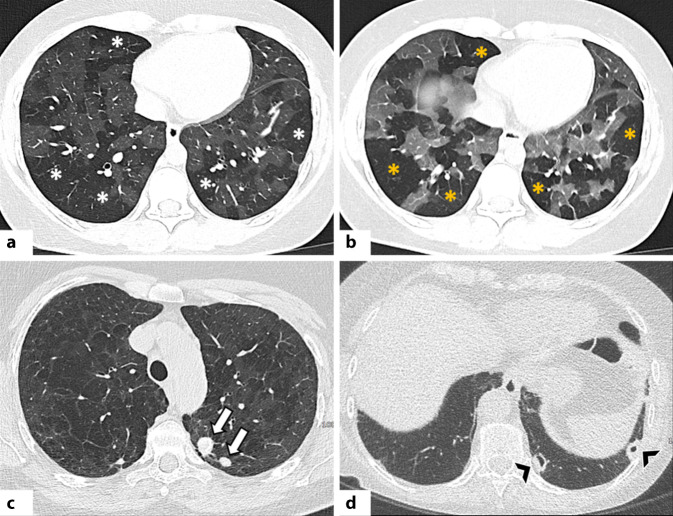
Obliterierende Bronchiolitis bei einer Patientin mit rheumatoider Arthritis (RA). a Axiale hochauflösende Computertomographie (HRCT) in Inspiration zeigt eine Mosaikperfusion bzw. -muster mit Arealen verminderter Dichte (*weiße Sternchen*) zwischen normal belüftetem Lungenparenchym. b In der Exspirationsaufnahme bestätigen sich diese Befunde als Areale von Air-Trapping (*gelbe Sternchen*). Rheumatoide Knötchen sind in c und d deutlich erkennbar. In c zeigen sich runde, periphere, solide Knötchen (*weiße Pfeile*) im apikoposterioren Segment des linken Oberlappens, während Bild d subpleurale, kavernierte Knötchen (*schwarze Pfeilspitzen*) im linken kostodiaphragmalen Winkel zeigt
